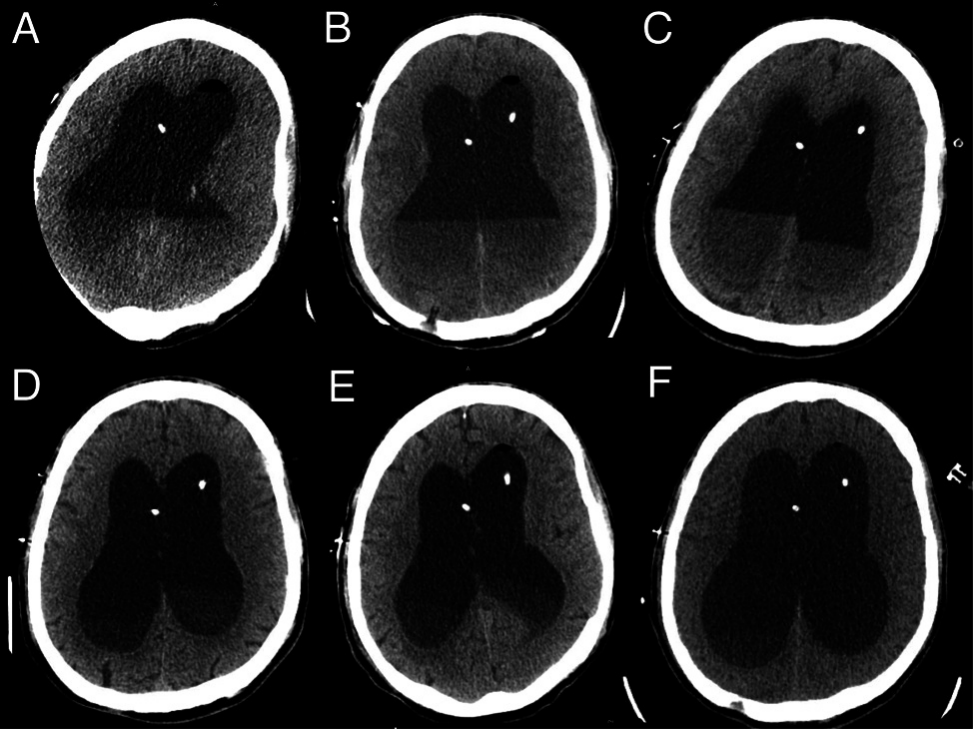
Axial non-contrast computed tomography (CT) cuts on treatment day 1, 2, 3, 7, 10, and 14 (A-F, respectively)
